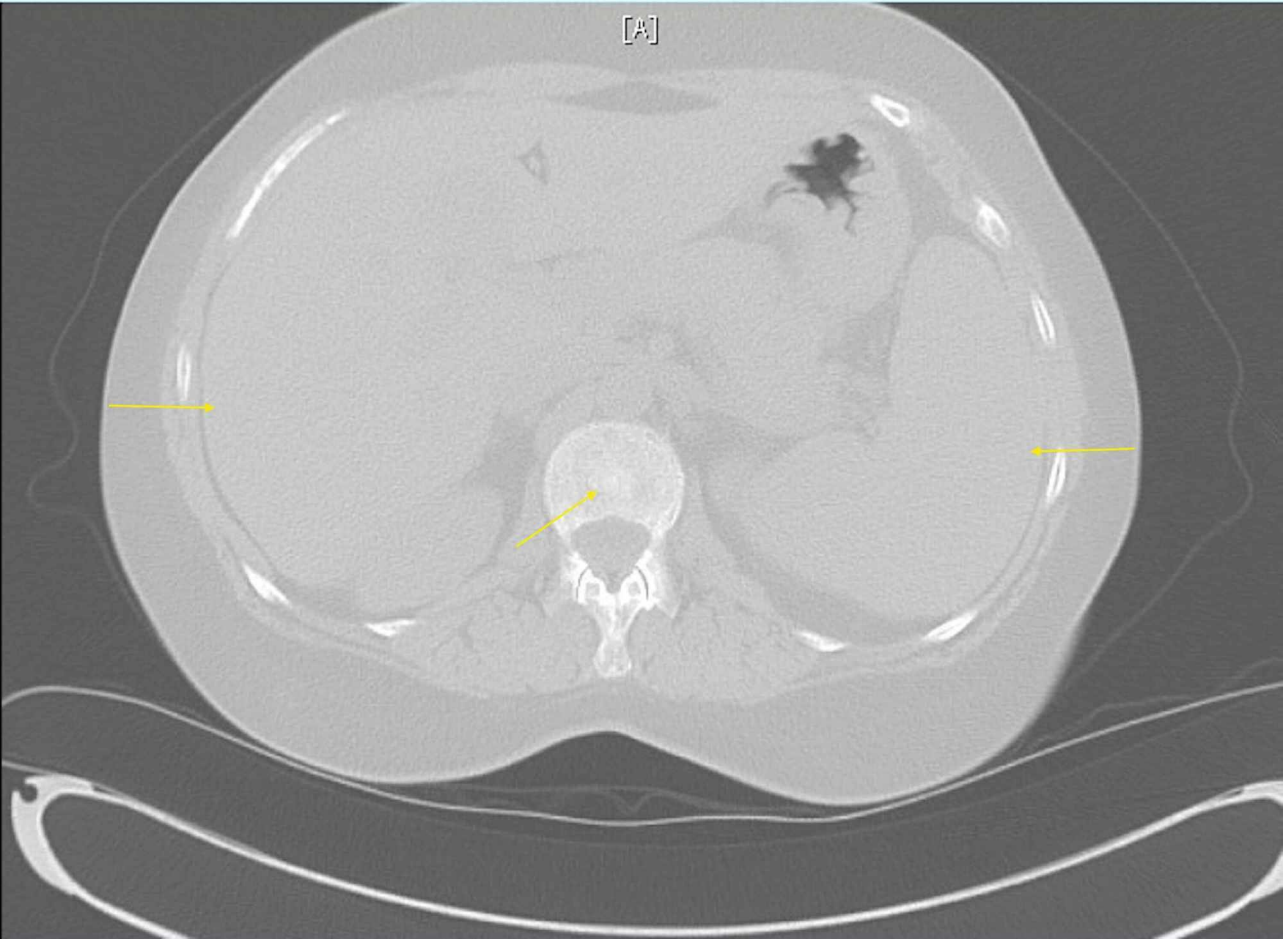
Figure 1: CT of the abdomen/pelvis without contrast Hepatosplenomegaly and patchy sclerosis of bones can be seen.
